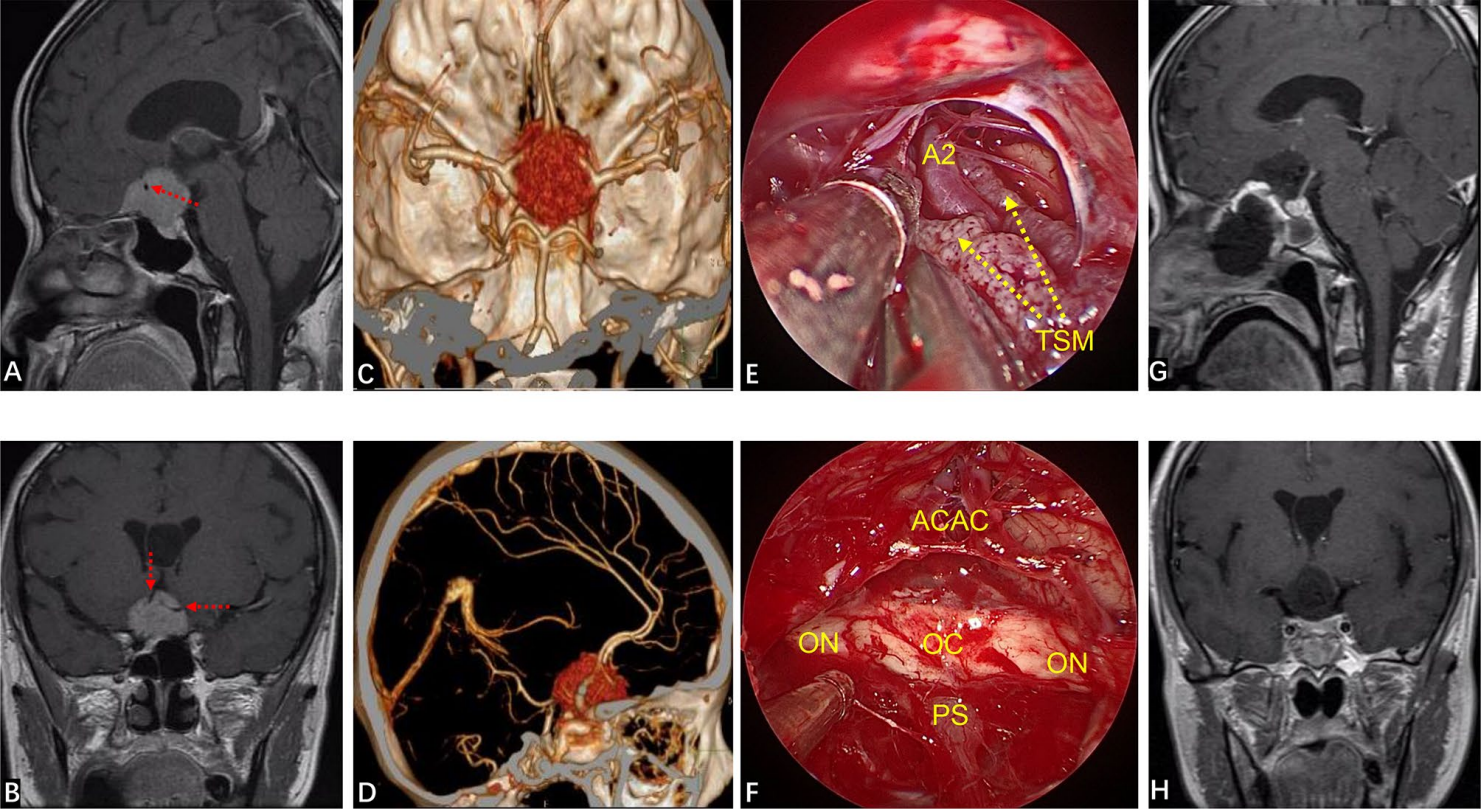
(A,B) Preoperative contrast-enhanced MRI of the sellar region showed the ACA (red dotted arrow) was wrapped by the tumor at 360°. (C,D) Preoperative computed tomography angiography (CTA) examination indicated that the tumor to be completely wrapped around the ACAC and was closely related to the ICA. (E) This intraoperative image shows the tumor to be completely wrapped around the A2 branch of the ACA. (F) After the tumor had been removed completely, the optic nerve, pituitary stalk and ACAC was well protected. (G,H) At POD3, contrast-enhanced MRI of the sellar region showed complete resection of the tumor and that the optic nerve, pituitary stalk and pituitary gland were intact.
